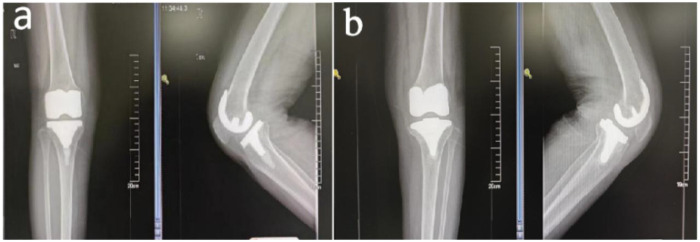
Preoperative anteroposterior and lateral X-rays of both knees: (a) right knee, (b) left knee.
